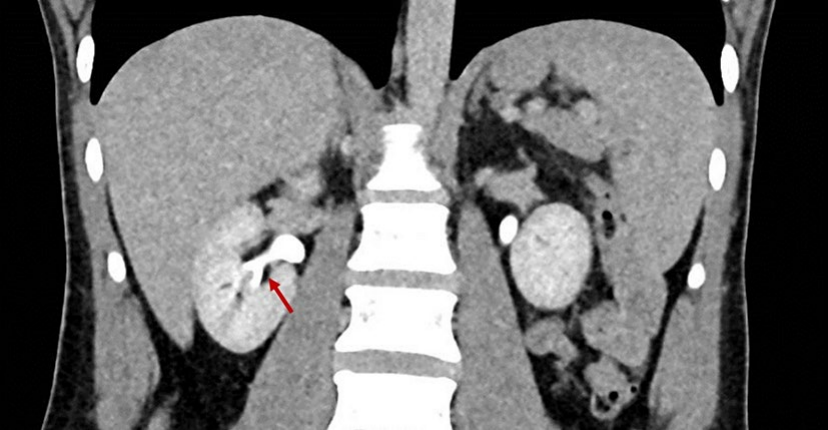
The sagittal view of the contrast-enhanced computed tomography image of the kidney shows the patient's kidney. A typical radiologic sign of renal papillary necrosis is the presence of necrotic cavities filled with contrast, along with the accumulation of contrast around the periphery of the calyces in the papillary region. This is called the "Ball-on-tee" sign (red arrow) [6].
